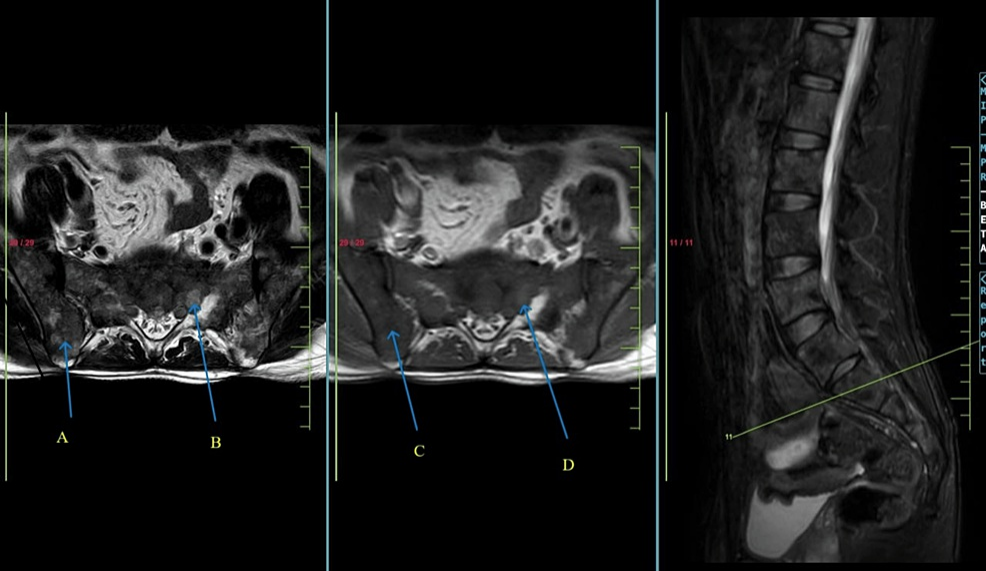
Magnetic resonance imaging (MRI) of the whole spine of the patient suggestive of minimal anterolisthesis of L5 over S1 with bilateral L5 pars interarticularis defects. (A) Pelvic bone heterogenous signal intensity in the T2 axial image, (B) sacrum bone heterogenous signal intensity in the T2 axial image, (C) pelvic bone hypointensity in the T1 axial image, and (D) sacrum bone hypointensity in the T1 axial image.
